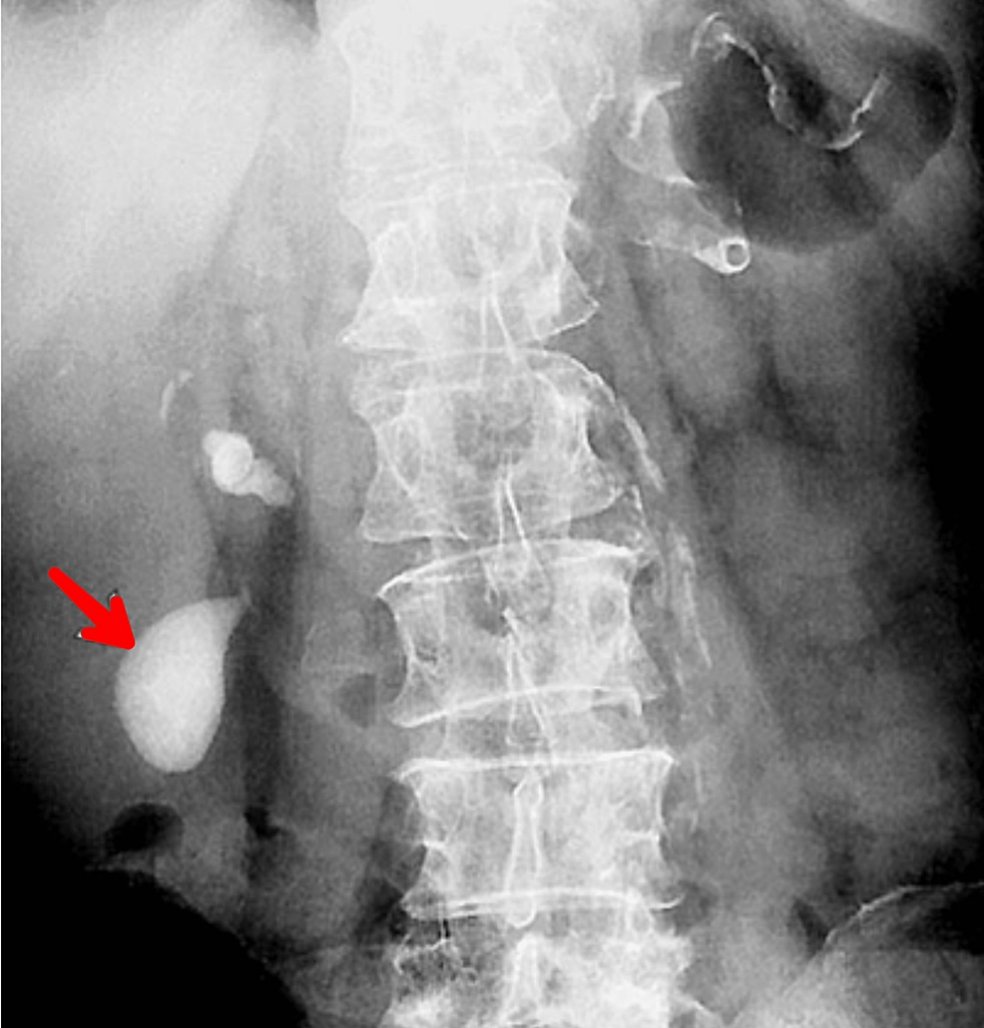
Abdominal x-ray showing a radio-opaque collection of secretions in the gallbladder.
